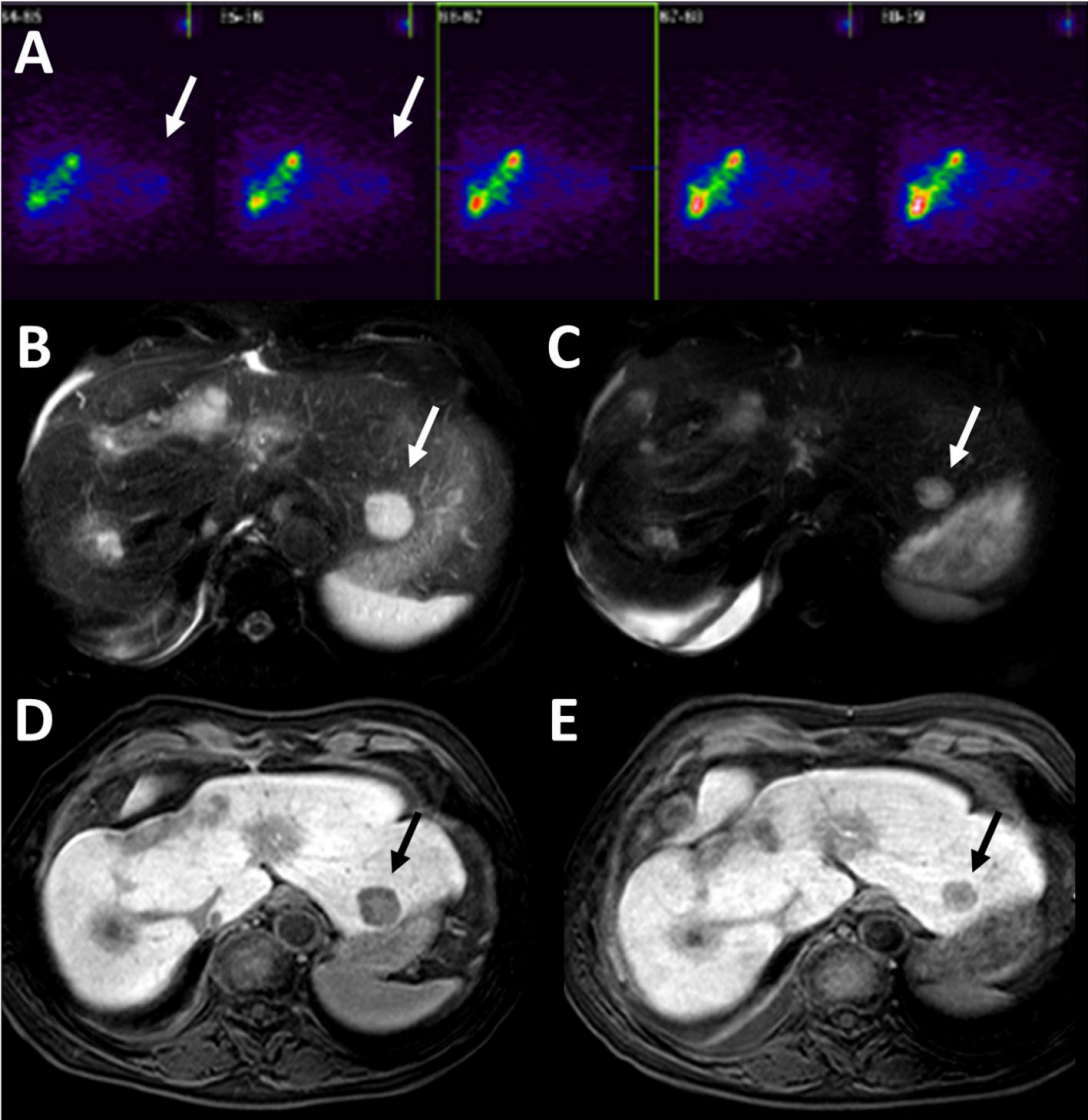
Patient B, prostate cancer, A-E, questionable abscopal effect: A SPECT (without CT): retrospectively uncertain whether there is some ^90^Y contamination on the left side (white arrow). B and C MRI1 and MRI2: Metastasis size regression (white arrow) in fat saturated T2w MRI. D and E MRI1 and MRI2: black arrow shows size regression in T1w 20 min after administration of primovist
